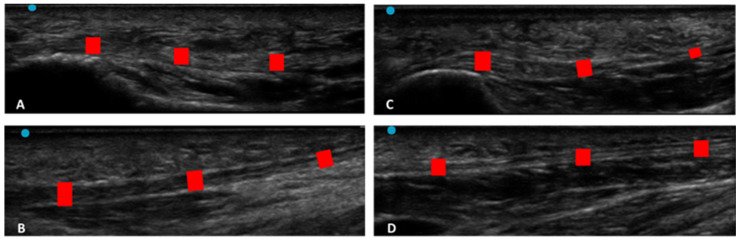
Ultrasound images of plantar fascia thickness: (A) group 1: PF level 1; (B) group 1: PF level 2; (C): group 2: PF level 1; (D) PF level 2. Red rectangles: plantar fascia.
